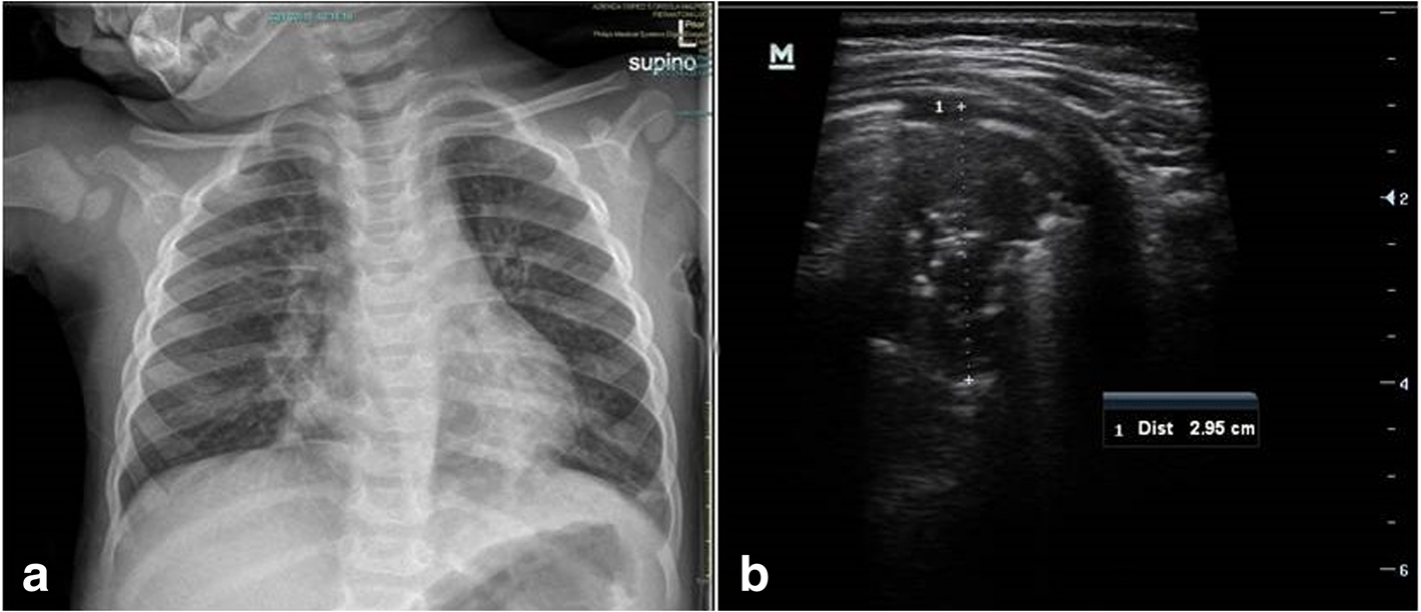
Comparison of CXR and LUS in a patient with bronchiolitis complicated by pneumonia in the left lung. a CXR demonstrated a basal left consolidation suggestive of pneumonia and bilateral peri-bronchial thickening. b Ultrasound showed a consolidation with air bronchograms in the posterior region of the left lung
